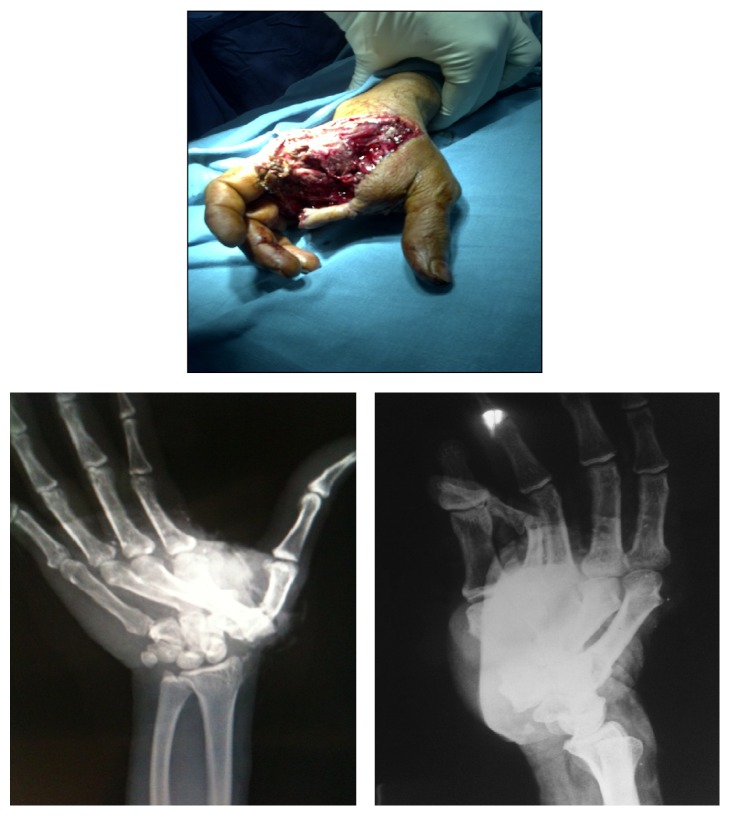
Clinical picture and X-ray at the time of injury.
